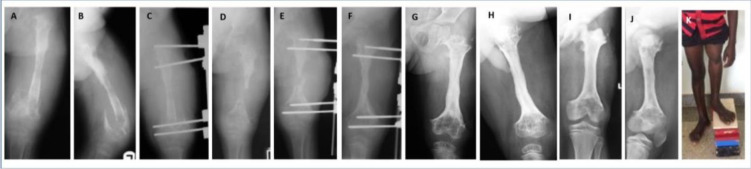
A 2-year-old child with a severe case of haematogenous femoral osteomyelitis presenting 2 months after the onset of symptoms. Radiographs showed sequestration of the entire diaphysis, pathological fracture and physeal involvement (a, b). Debridement and stabilization with an external fixator were performed, leaving the sequestrum in situ (c). This allowed better demarcation of the sequestrated segment and time for development of periosteal involucrum. This is common in small children. Sequestrectomy was performed 3 months later. Ten months later, the child presented with a bone defect in the mid-femur without any sign of infection (d). A tibial cortical strut graft was placed and secured by trans-osseous stitches. An external fixator was reapplied (e). Sound integration of the graft was observed at 16 weeks (f) and the fixator was removed. Radiographs are shown 1 year after the graft procedure (g, h). Graft hypertrophy and cylindrical transformation of the graft were noted, together with early closure of both physes, deformity of the femoral condyles and patella baja. An orthoprosthesis for the leg length discrepancy (LLD) was fabricated. At 8 years, he was walking without aids or a shoe raise. He had a 17 cm LLD and no infection relapse. The radiographs showed a short femur, knee deformity and patella baja (i, j, k).
